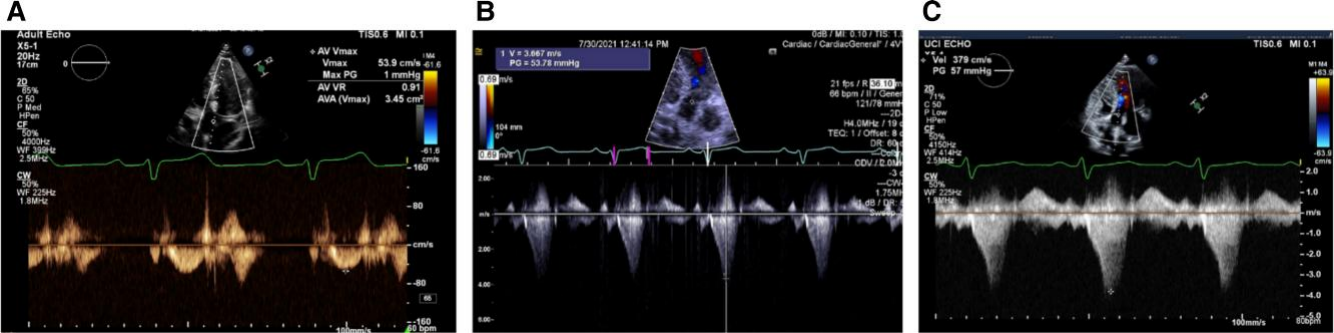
Serial echocardiograms demonstrating no left ventricular outflow gradient on admission (*A*) and dynamic peak gradients after fluid resuscitation and verapamil discontinuation on hospital Day 2 (*B*) and prior to discharge (*C*).
